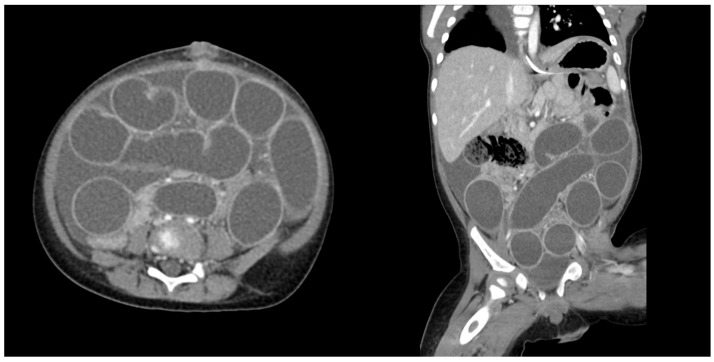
Postoperative computed tomography scan on a 14-month-old patient after water bead ingestion, prior to second look operation. Dilated loops of bowel visualized without distinct beads seen.
